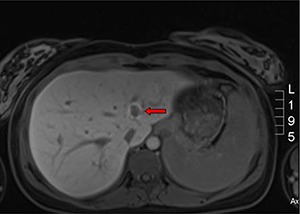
T1 weighted MRI (axial view): Liver lesion consistent with focal nodular hyperplasia (arrow).
